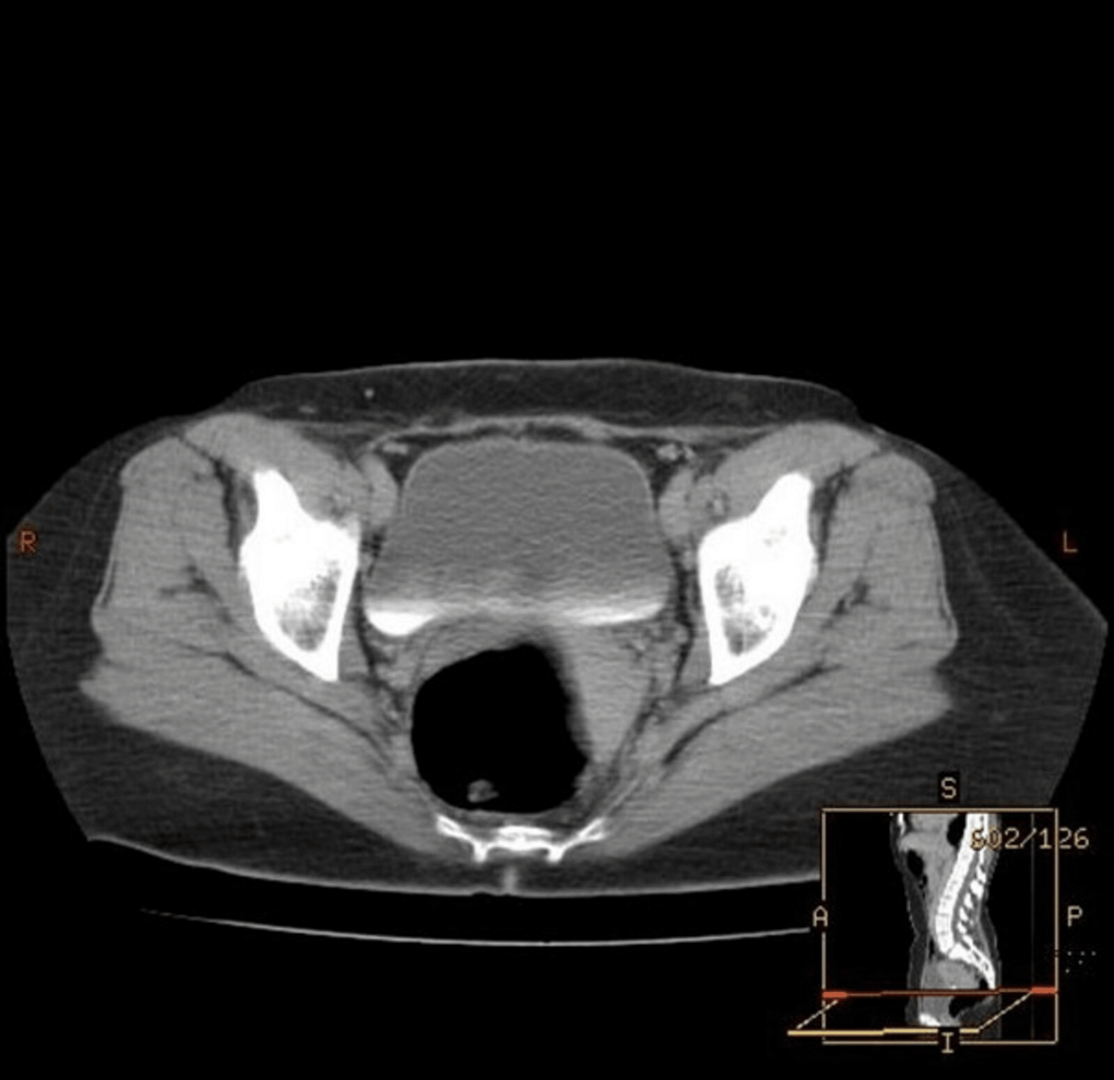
CT of the pelvis (axial view) demonstrated normal bladder with no signs of inflammation.
